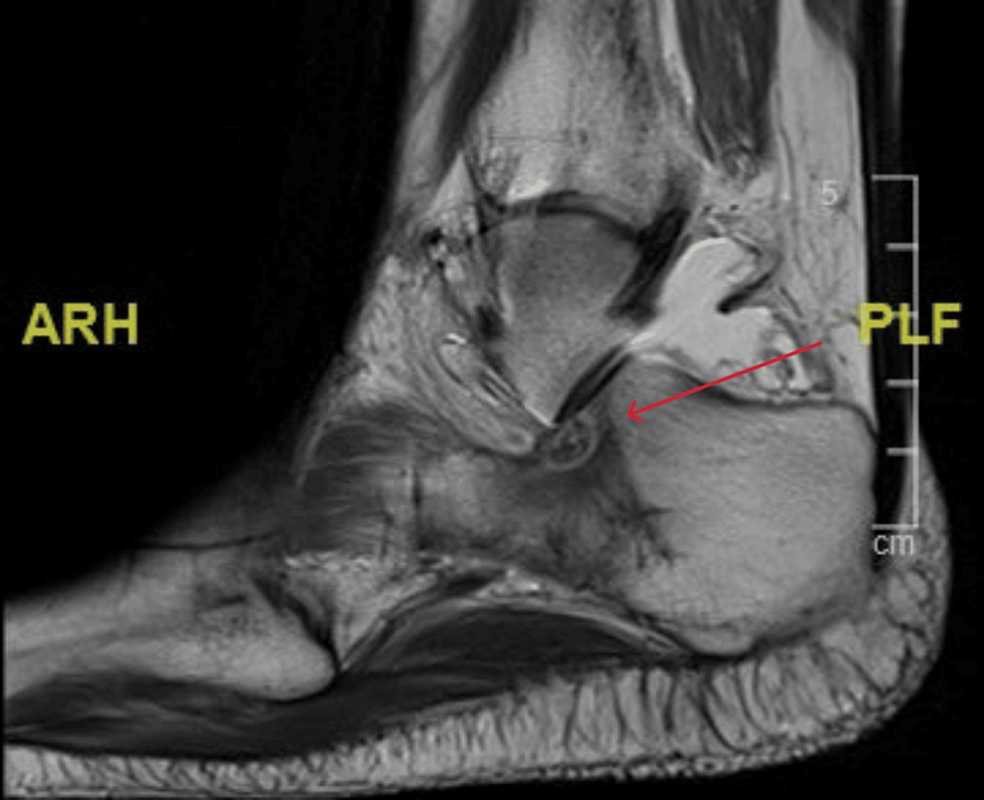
Sagittal view MRI T1 window of the right ankle at the time of presentation MRI: magnetic resonance imaging
